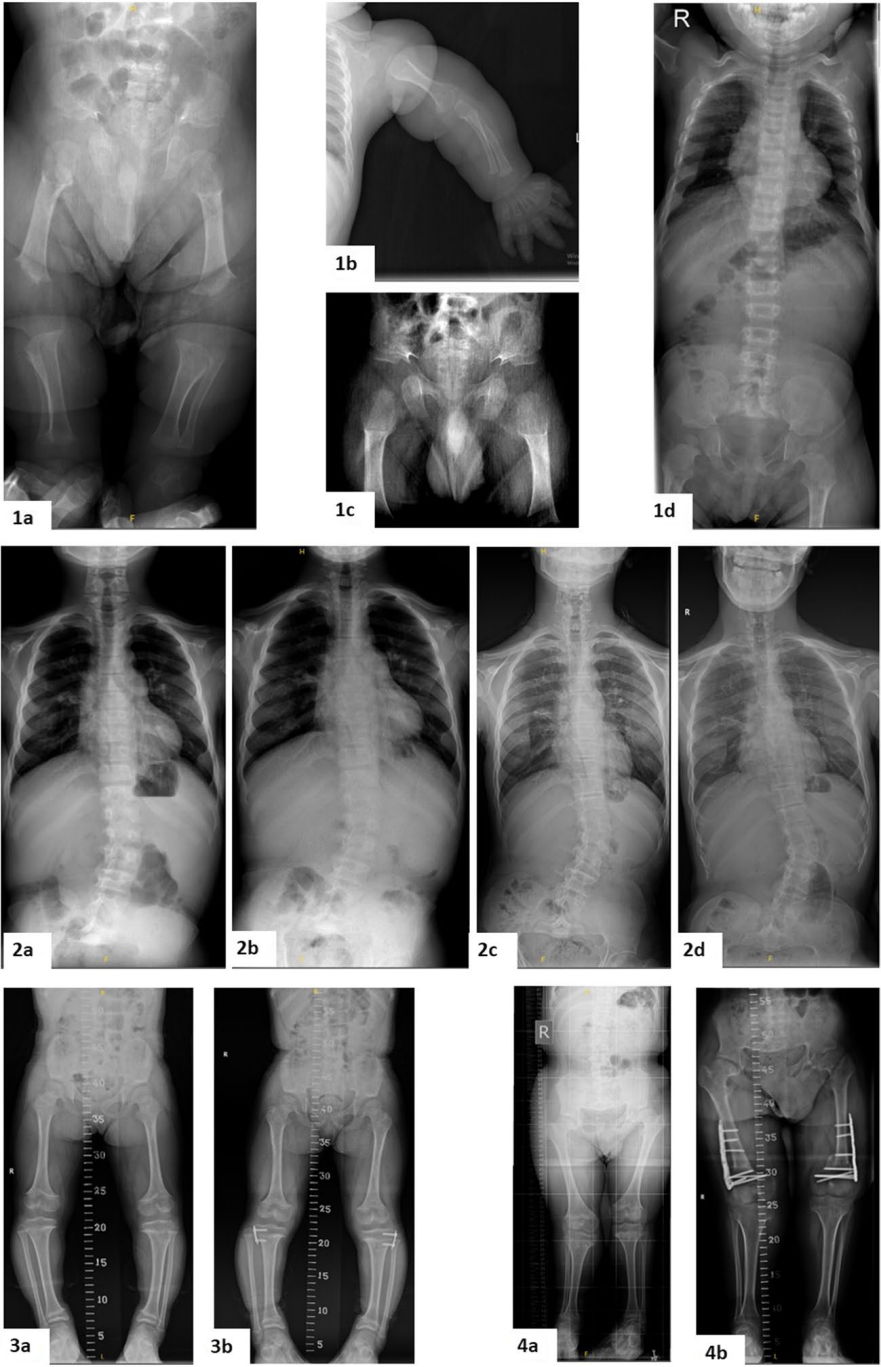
The typical radiographic findings in ACH. Please note; 1a) generalized metaphyseal changes and proximal femoral radiolucency; 1b) short tubular bones, rhizomelic shortening of the arms, and trident hand; 1c) proximal femoral radiolucency and narrow sacrosciatic notch; 1d) narrowing of the interpedincular distance of the caudal spine in infancy period; 2a-2d) progressive scoliosis in a patient aged 6, 9, 16, and 19 years; 3a-3b) genu varum in a patient and postoperative radiograph aged 8–9 years; 4a-4b) genu valgum in a patient and postoperative radiograph aged 15–17 years
